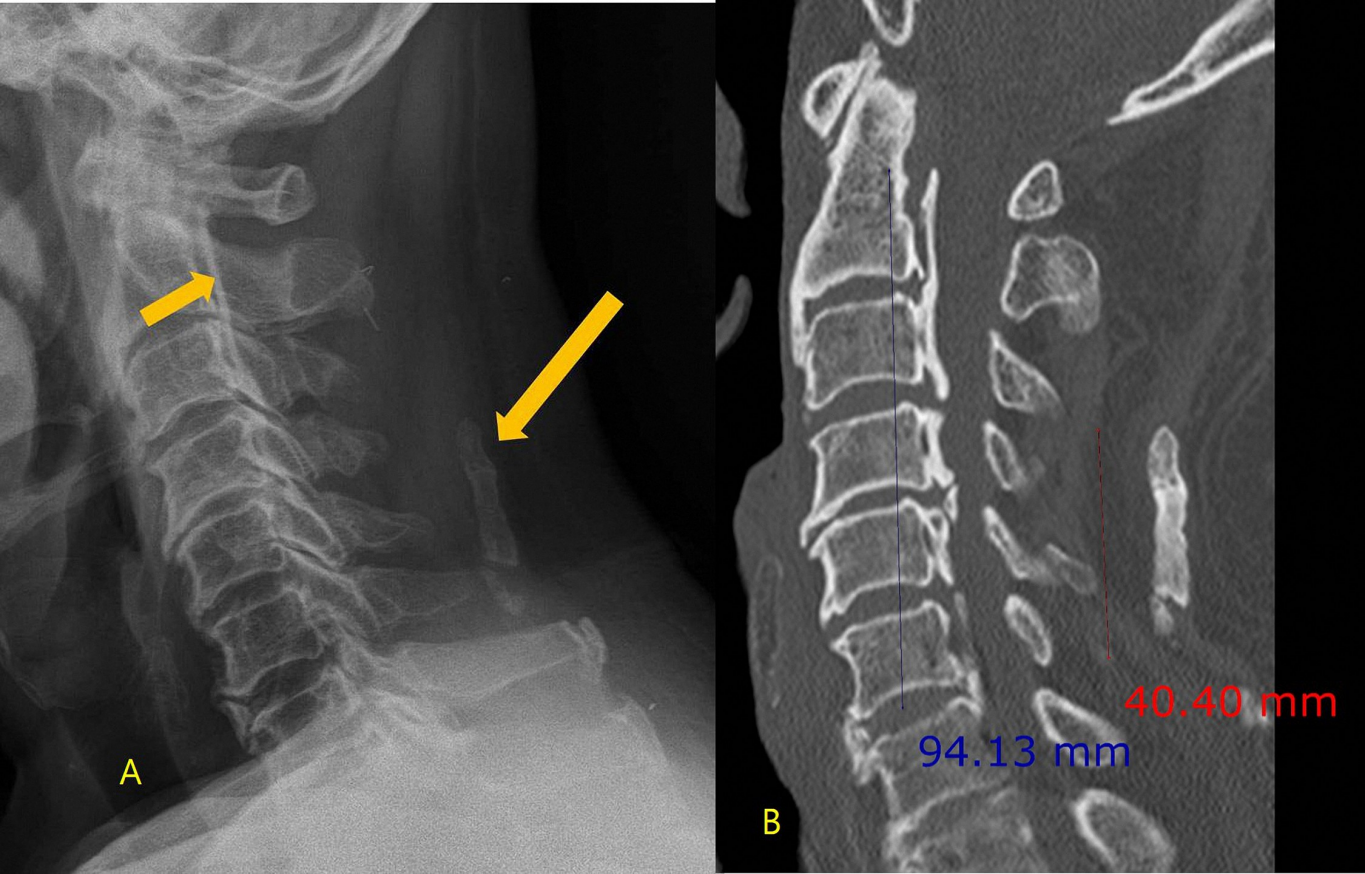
70-year-old man with neck pain after fall down. (A) Lateral radiograph of the cervical spine showing a linear increased density on the posterior surface of the vertebral bodies (short arrow) and ossification of the nuchal ligament (long arrow). (B) The sagittal reconstructed CT image revealed ossification of the posterior longitudinal ligament (long double arrow) and nuchal ligament (short double arrow).
